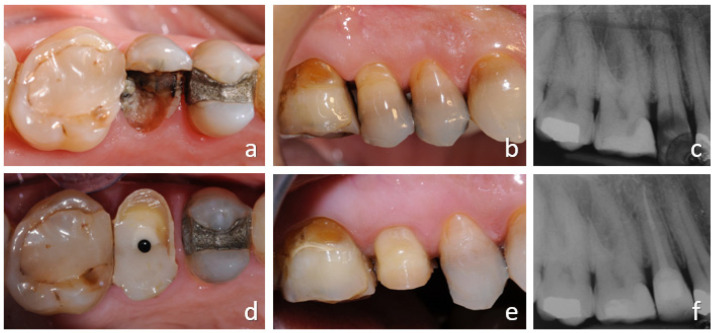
Orthodontic extrusion of tooth 1.5 with insufficient ferrule effect. Preoperative clinical occlusal and vestibular views and radiograph (a–c): tooth with deep caries. Endodontic treatment and post-endodontic restoration with post was performed: occlusal and buccal views and post-operative radiograph (d–f).
